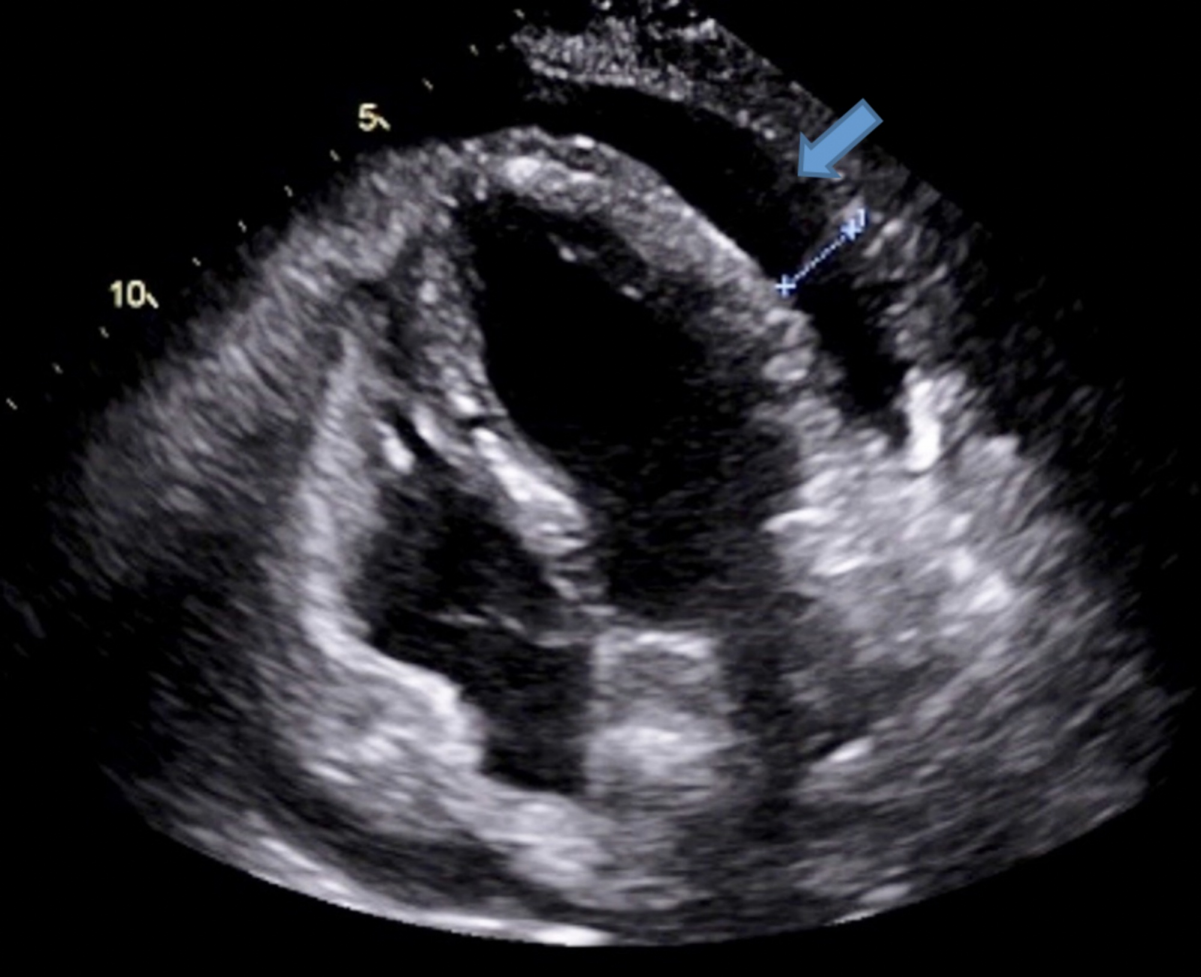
Apical 4 chamber view showing an interval increase in the size of the effusion.
